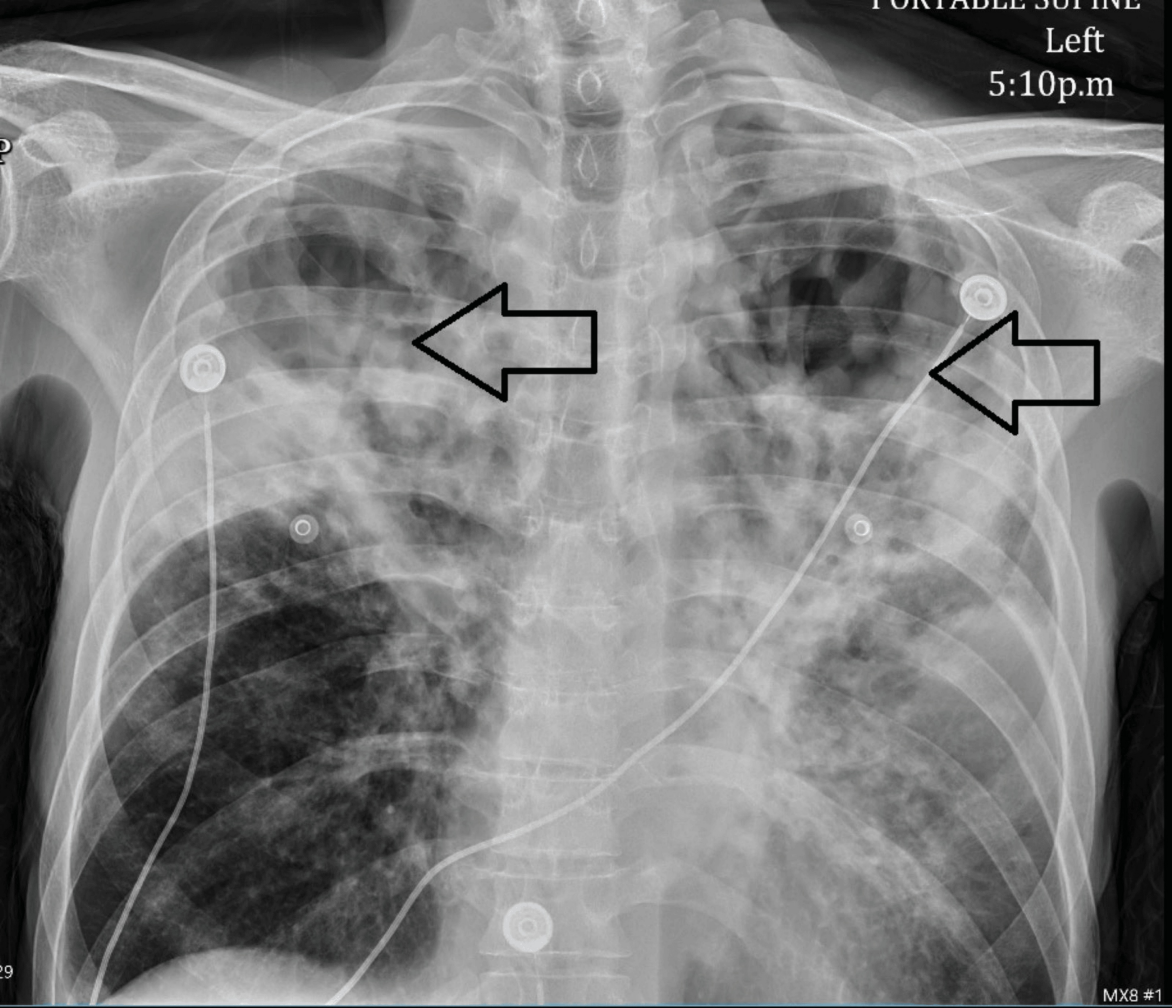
Chest X-ray showing cavitary lesions (black arrows)
